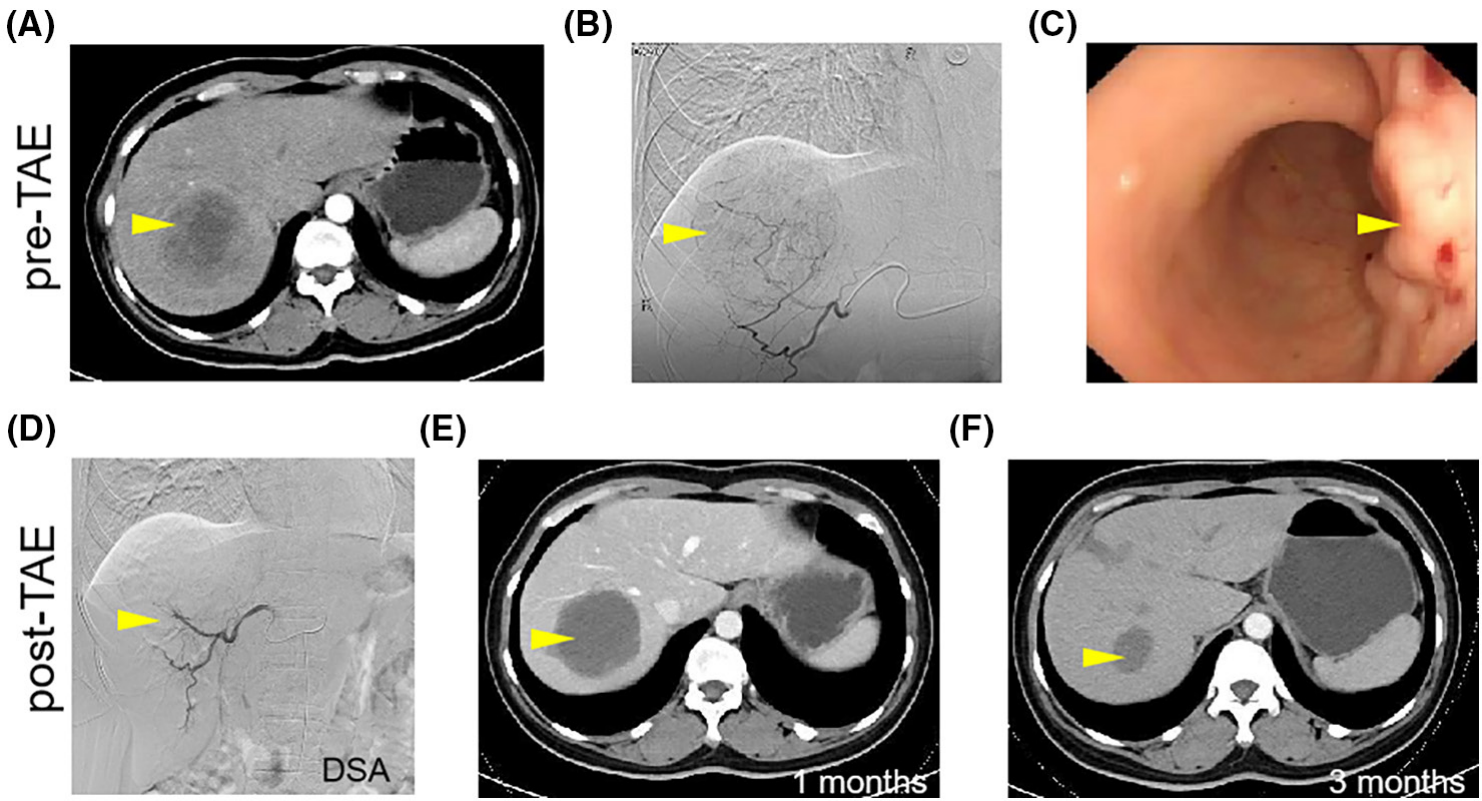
Effect of TAE therapy in a patient with rectal NENLM. (A, B) A patient with rectal NENLM type III with hypovascularism was evaluated by contrast‐enhanced CT and DSA before TAE. (C) Primary rectal NENs were observed under endoscopy. (D, E) The main blood vessels of patients with NENLM were blocked, allowing the tumour to liquefy, necrotic, and shrink. (F) The tumour had shrunk significantly 3 months later by CT examination. Yellow arrows indicated NENLM lesions. CT, computer tomography; DSA, digital subtraction angiography; NEN, neuroendocrine neoplasm; NENLM, neuroendocrine neoplasms with liver metastasis; TAE, transhepatic artery embolization.
